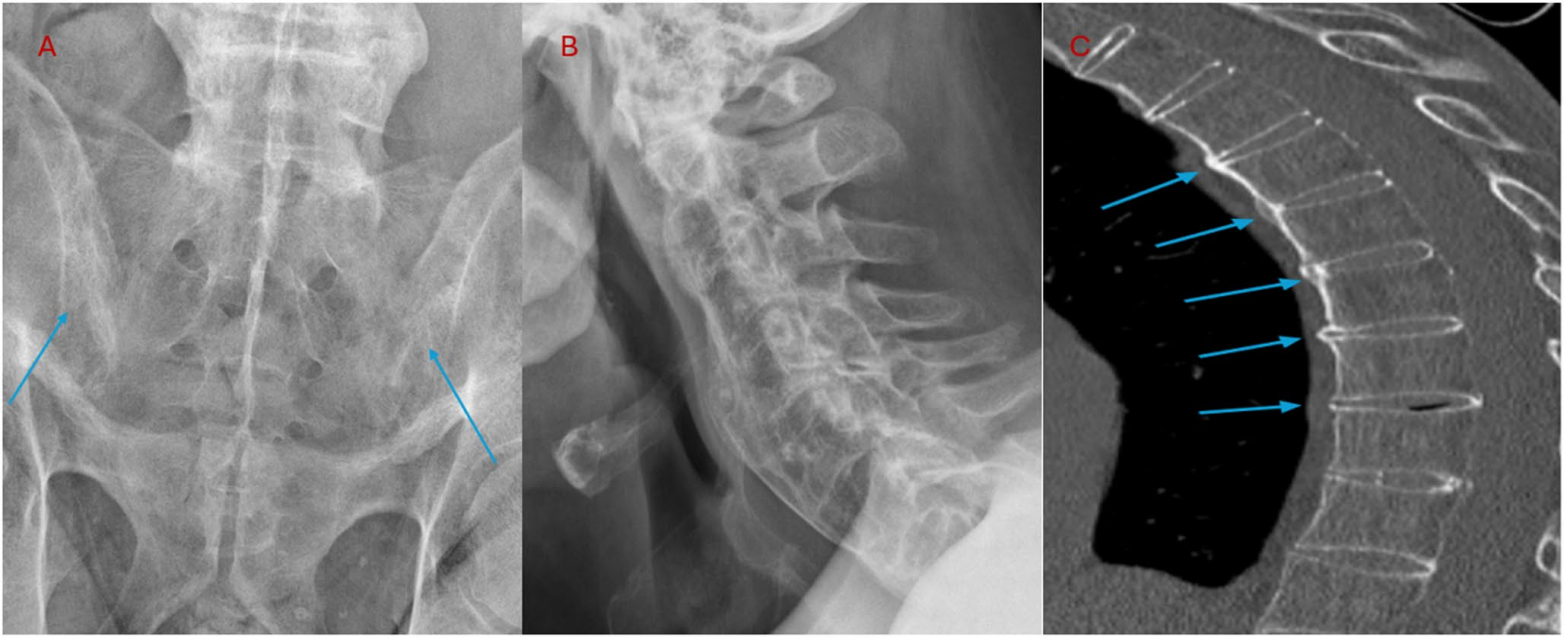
A Pelvic radiograph, anteroposterior view: sacroiliac joint changes consistent with ankylosing spondylitis (axial spondyloarthritis). B Cervical spine, lateral radiograph: complete fusion of anterior and posterior spinal elements, producing the classical “bamboo spine” appearance. C Thoracic spine, sagittal CT: thin vertical syndesmophytes are visible (blue arrows) in female patient with axial spondyloarthritis
